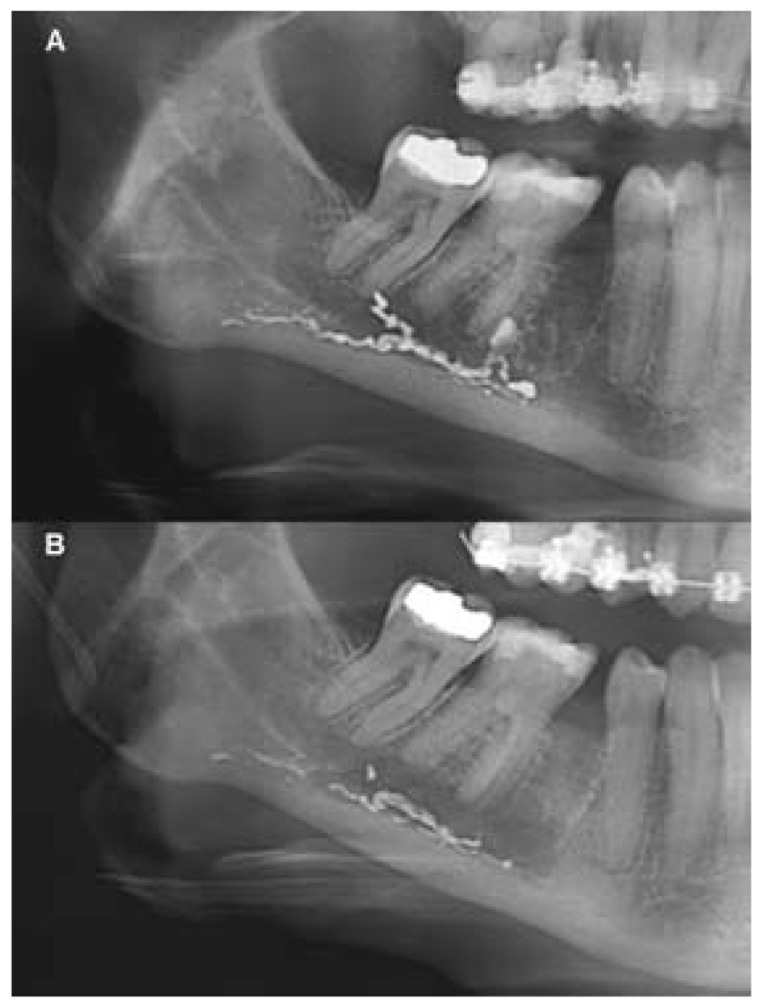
A) Initial panoramic radiograph detail. B) Panoramic radiograph detail after three weeks of treatment. A significant reduction in the amount of endodontic sealer within the mandibular canal is observed.
